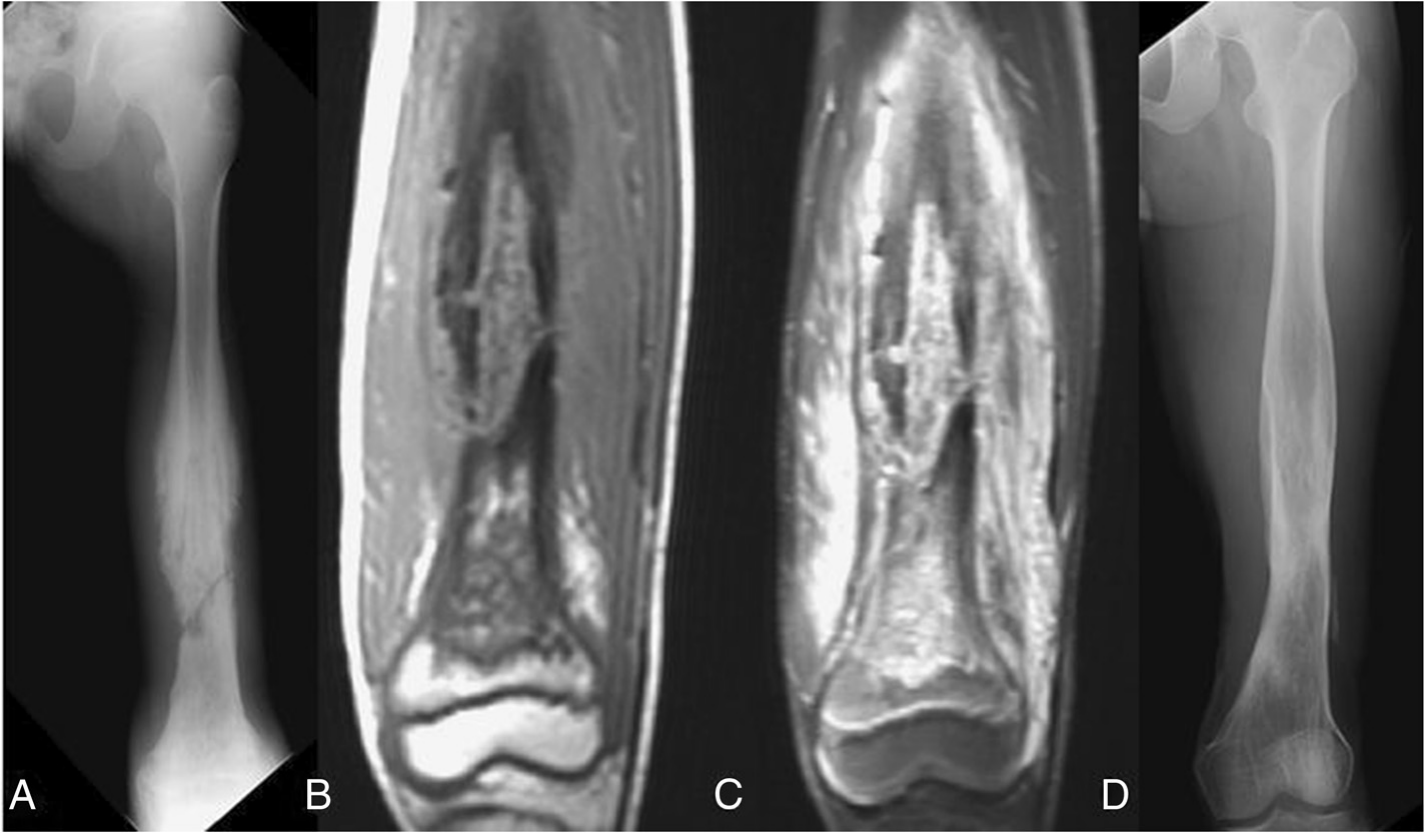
A 14-year-old boy who experienced moderate progressive thigh pain, especially at night, for four months. (A) A plain film of the femur showing a laminated periosteal reaction with pathological fracture at the distal femur. (B, C) Magnetic resonance imaging (MRI) showing tumor-like lesion at the marrow surrounded with extensive soft tissue edema. (D) A plain film of the femur taken two years after casting and debridement surgery showing bony union.
